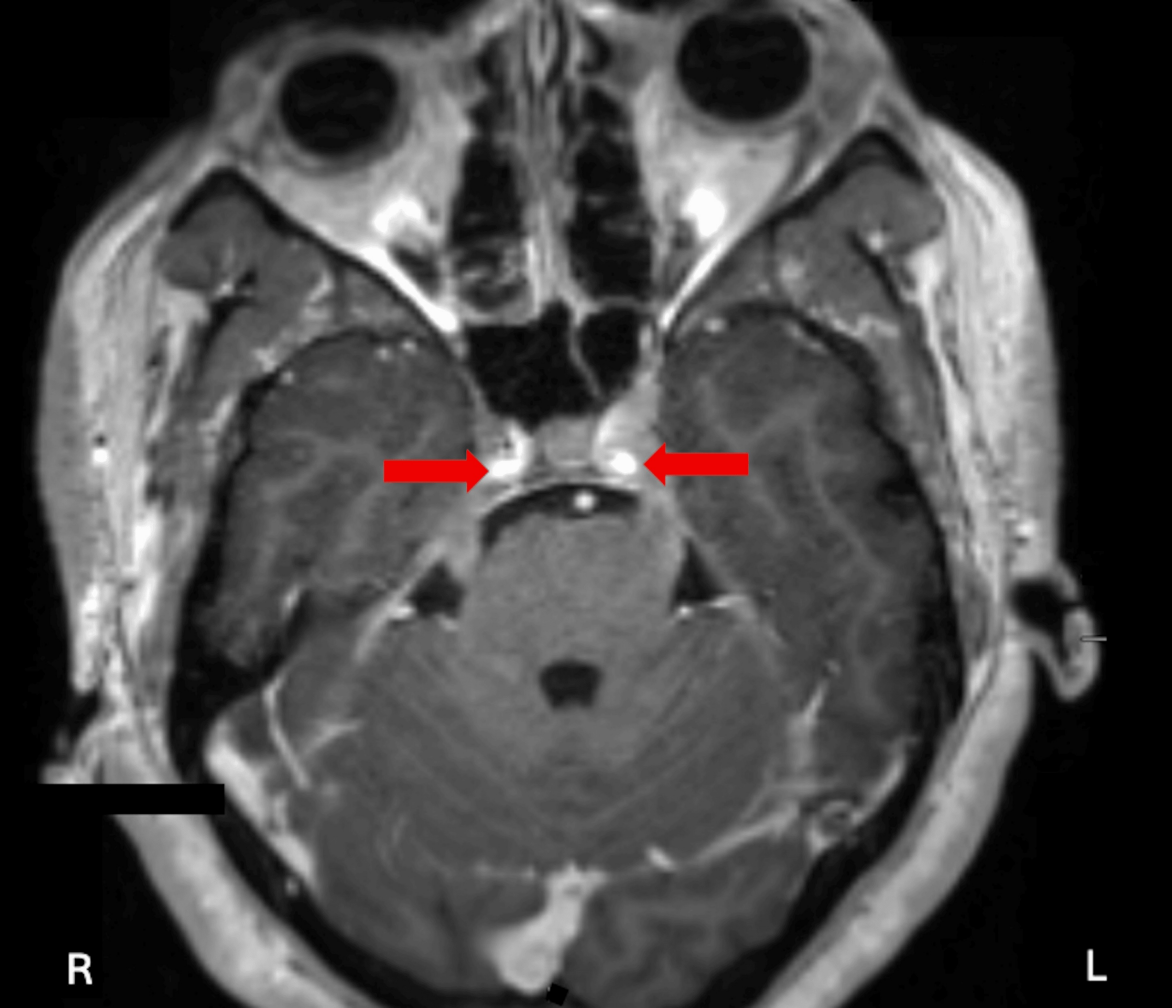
Axial post-contrast T1-weighted magnetic resonance imaging (MRI) of the brain demonstrates abnormal leptomeningeal enhancement along multiple cisternal cranial nerves with extension into the bilateral Meckel’s caves (red arrows).
